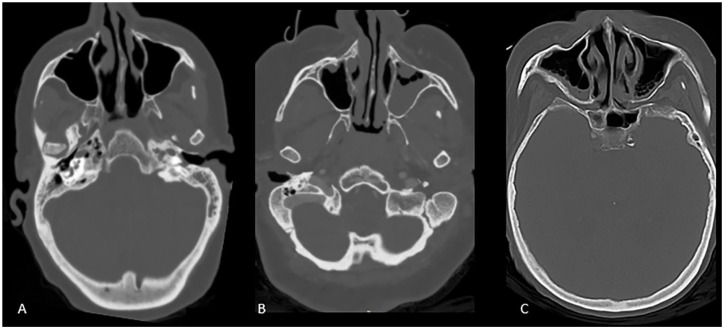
Sinus opacification grades. Axial CT scan images demonstrate normally aerated
